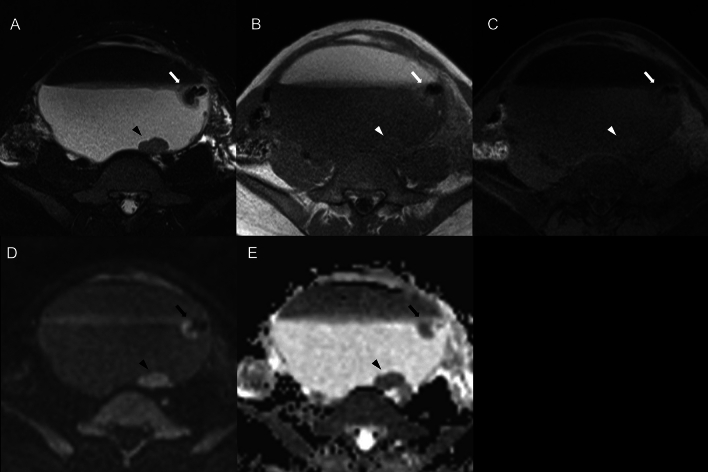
A 25-year-old woman with squamous cell carcinoma arising in an ovarian cystic teratoma without transmural extension. MR images show two distinct mural nodules: one consistent with “findings suggestive of malignancy” (mural nodule with diffusion restriction and without fat content, calcification, or Palm tree appearance) (arrowhead) and another exhibiting calcification and fat content (arrow). a Axial fat-suppressed T2-weighted image reveals a 155 mm-sized large cystic lesion in the pelvis. The nodule located dorsally in the cyst (arrowhead) shows isointensity, forming an acute angle with the cystic wall, while the nodule on the left side wall of the cyst (arrow) has strong hypointense areas suggestive of calcification. Neither nodule shows a Palm tree appearance. b, c In axial T1-weighted in-phase and fat-suppressed images, the nodule located dorsally in the cyst (arrowhead) is not clearly delineated. Conversely, the nodule on the left side wall of the cyst (arrow) presents areas of signal drop in the fat-suppressed images, indicating the presence of fat. d, e Axial diffusion-weighted images and ADC maps show that the nodule located dorsally in the cyst (arrowhead) has diffusion restriction. In contrast, the nodule on the left side wall of the cyst (arrow) has areas believed to be fat, with no other signs of diffusion restriction
